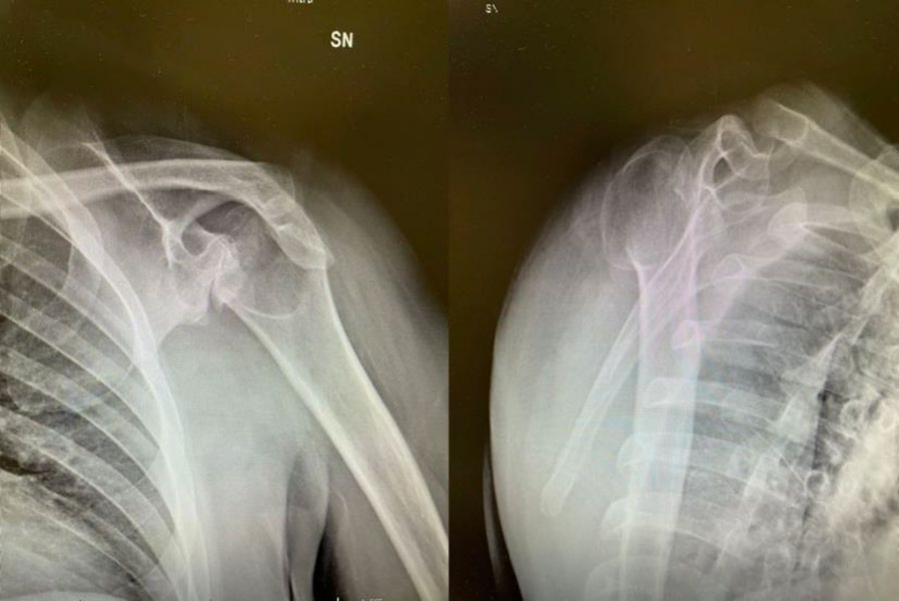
Antero-posterior and “Scapular-Y” Xrays views of OBPI shoulder in this study
